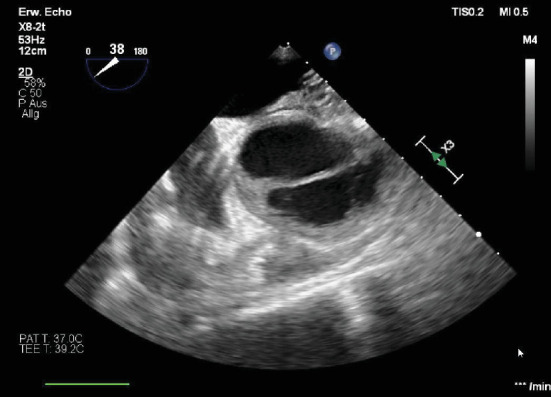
Preoperative transesophageal echocardiography (TOE) of the aorta in the short axis, with a visible dissection membrane and mural hematoma.
